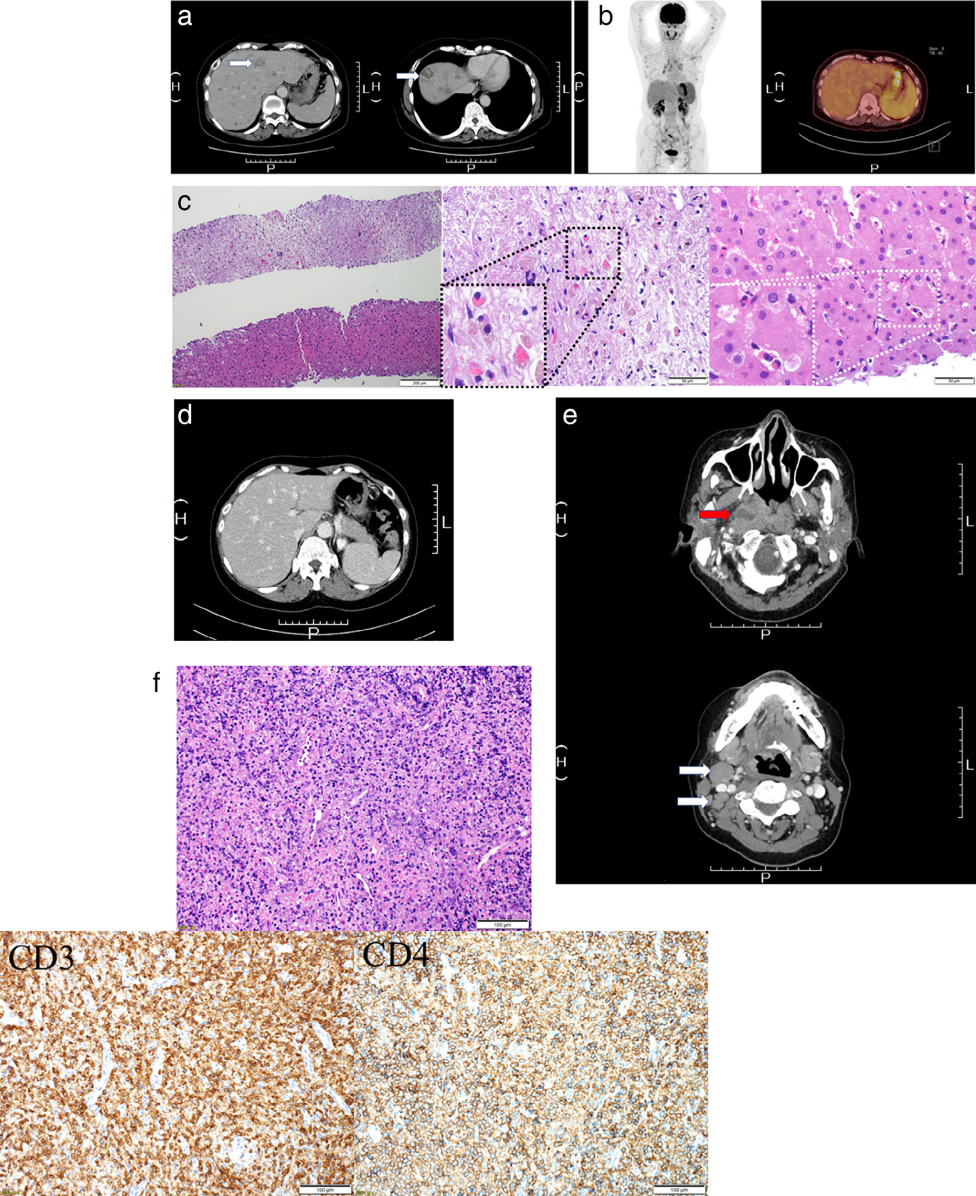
Computed tomography (CT) shows multiple hypovascular tumors (a; white arrows). Positron emission tomography‐CT could not detect primary tumor (b). Liver biopsy showed that the tumor consisted of scar tissue with remnants of eosinophil infiltration (c). Multiple liver tumors disappear after treatment (d). Ten months later, a hypovascular tumor in the nasopharynx and oropharynx (red arrow) and swelling of the surrounding lymph nodes (white arrows) appeared (e). Pathological analysis showed that the tumor was a T‐cell malignant lymphoma (f).
